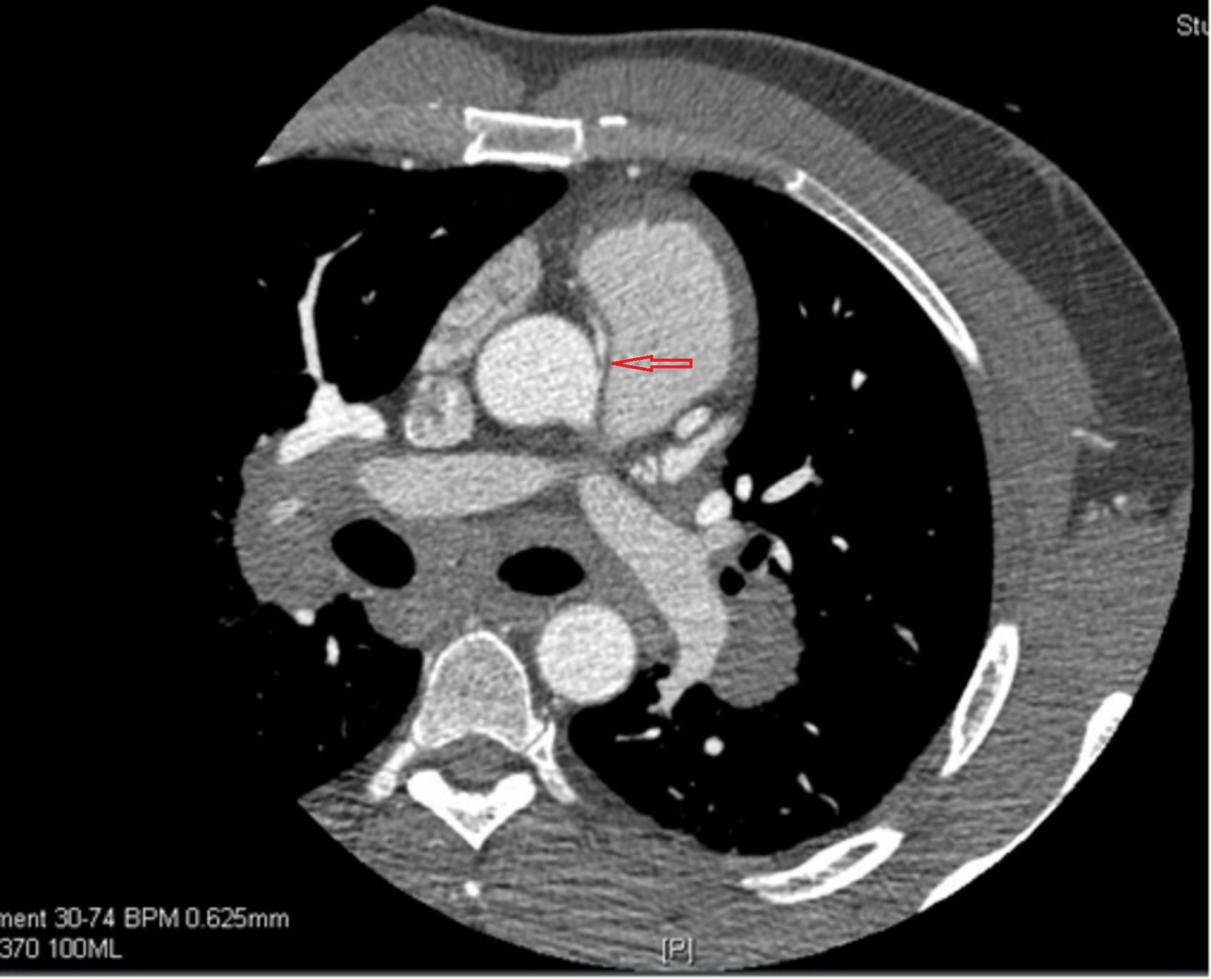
CCTA shows ARCA coming off the left coronary sinus, with slit like ostium (severe proximal right coronary artery narrowing). Red arrow points to ARCA. ARCA: Anomalous right coronary artery; CCTA: Coronary computed tomography angiography.
